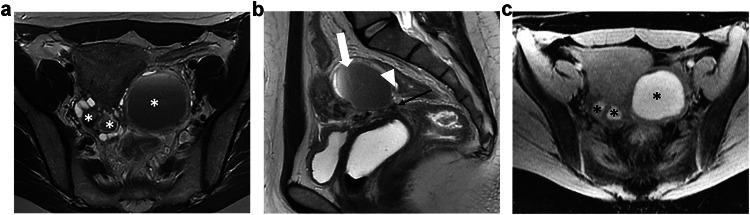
Bilateral ovarian endometriomas and kissing ovaries in a 35-year-old woman with severe pelvic pain. a Axial T2W MR image shows shading within the bilateral endometriomas (*). The ovaries are medially displaced in the retrouterine region. b Sagittal T2W MR image shows shading and fluid-fluid level within the left ovarian endometrioma (white arrow). There is also a low signal intensity thickening of the peritoneum of the ovarian fossa (black arrow) and tethering of the bowel serosa to the ovarian capsule (arrowhead). c Axial T1WFS MR image shows bilateral high-signal intensity cysts, likely due to hemorrhagic content (*)
